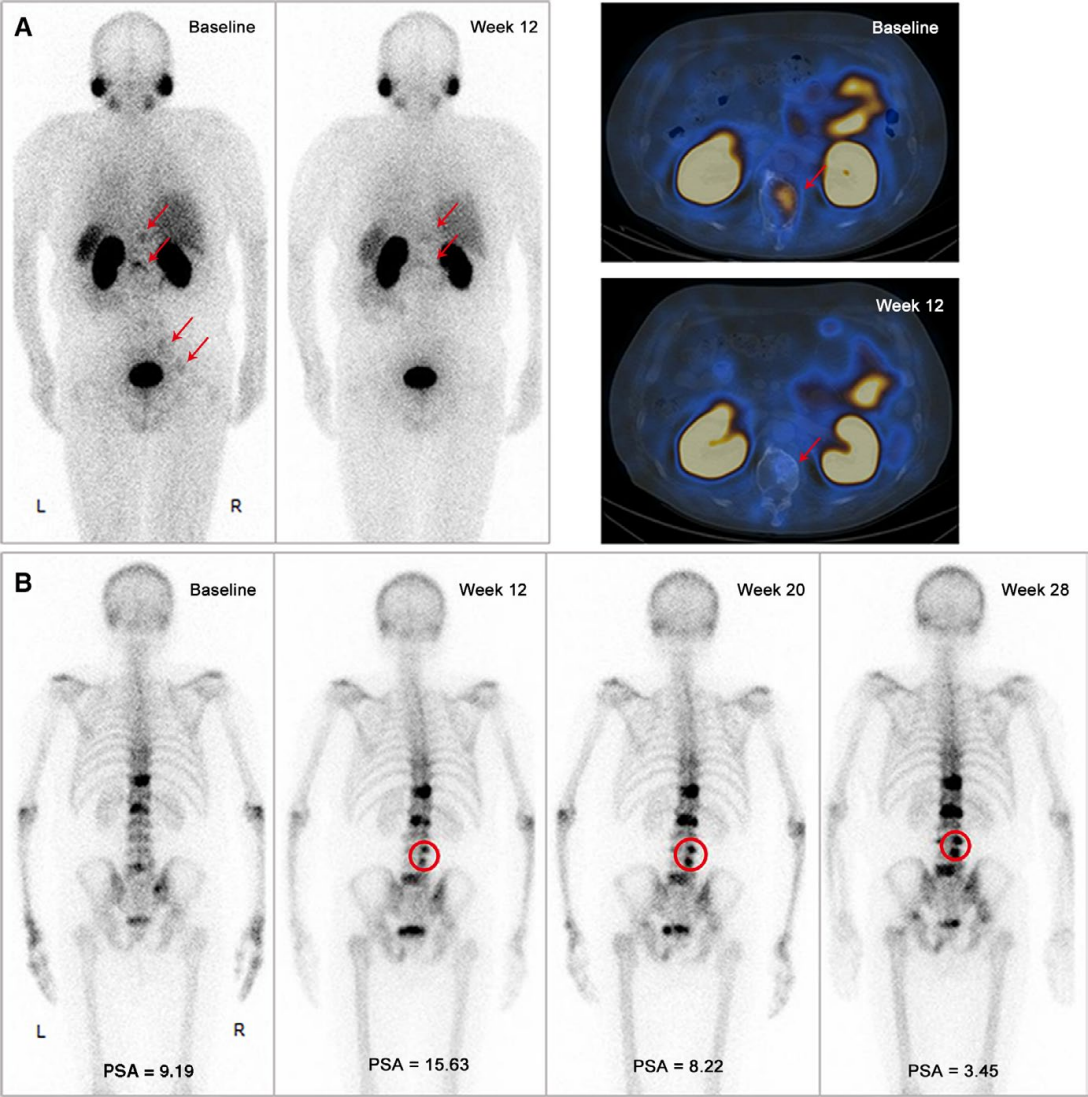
A 70‐yr‐old mCRPC patient with Gleason 4 + 5 who progressed after 20 months on traditional hormone treatment, and 8 docetaxel cycles of chemotherapy. PSMA imaging both before and after 12 weeks of abiraterone treatment showed a decrease in the miPSMA score of the bone lesions (A, red arrow). However, at week 12, there was a significant increase in the PSA and 2 new lesions on the bone scan (B, red circle). By weeks 20‐28, the PSA progression improved, and no additional lesions appeared on the bone scan, indicating that the progression seen at week 12 was due to PSA and a bone flare
